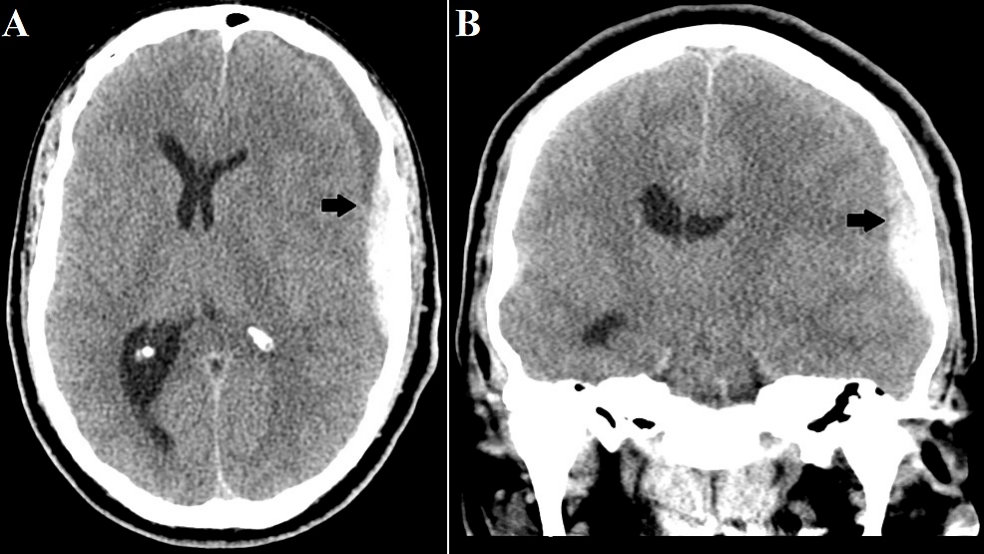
Preoperative computed tomography scan of the patient’s head demonstrating a 10 mm heterogeneously dense subdural hematoma (black arrow) with 10 mm of midline shift in axial (A) and coronal (B) planes.
